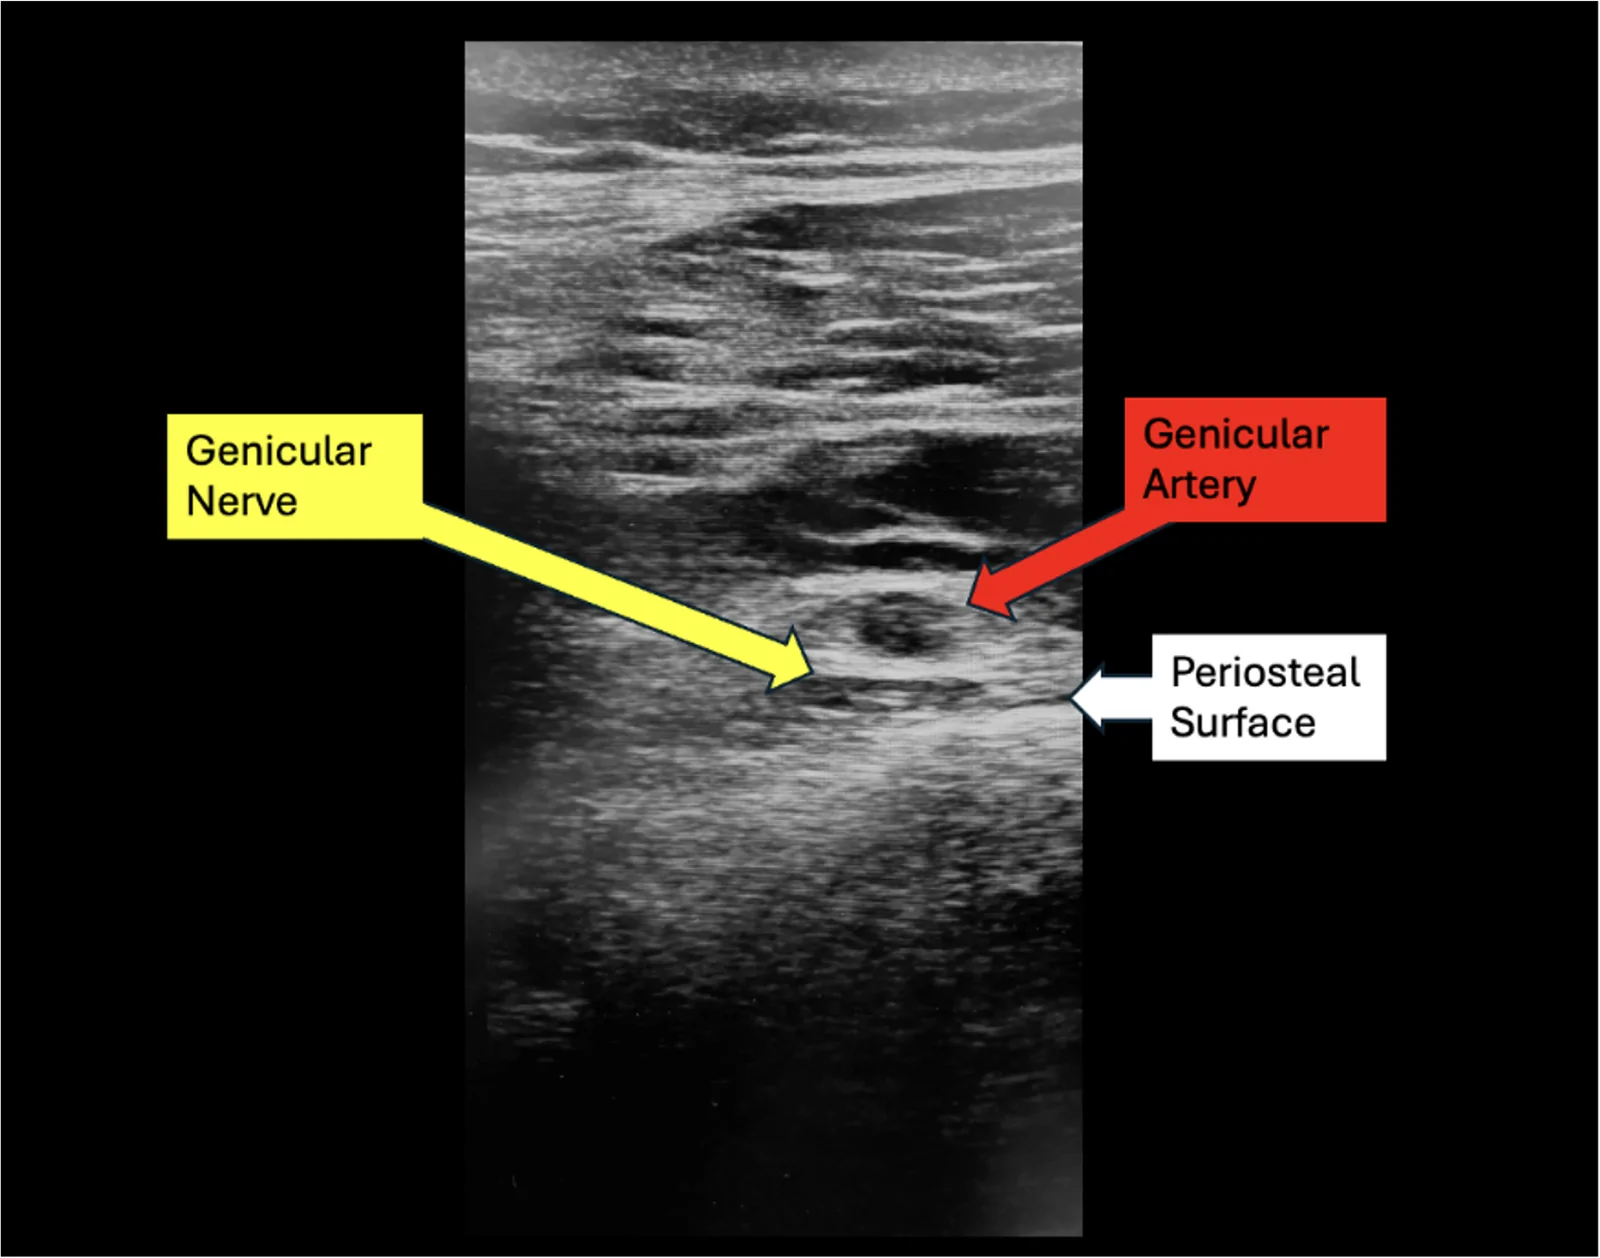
Genicular nerves are relatively easy to target with ultrasound. Highlighted are a genicular nerve, genicular artery, and the periosteal surface (original figure)
